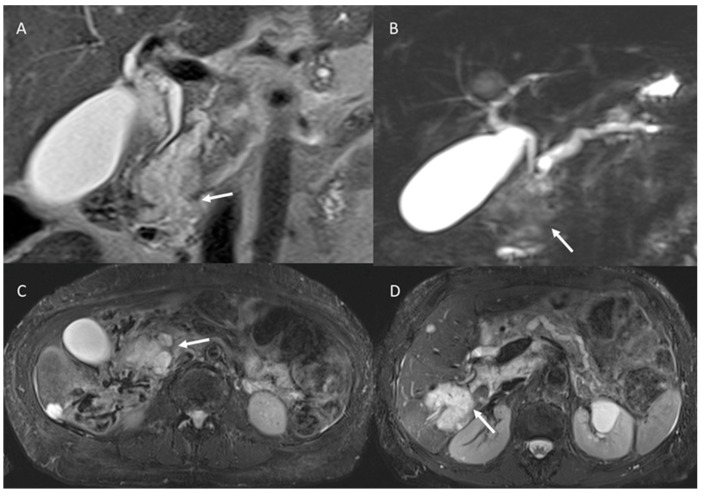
MRI assessment of degenerated MD-IPMN. In T2-W (A), MRCP (B) and T2-W FS (C) sequences the lesions appear (arrows) as a dilated MD with mucinous intraluminal component (arrow). In (D) (T2-W FS) arrow shows mucinous liver metastasis.
